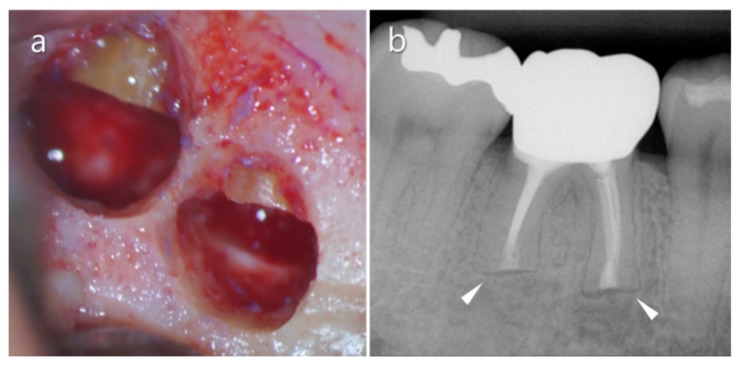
A contemporary technique for root resection. (a) Clinical features of mesial and distal roots resected perpendicular to the long axis of each root. (b) A periapical X-ray image taken using the parallel technique shows resection with a shallow bevel. Note that complete bony healing occurred with continuous lamina dura.
